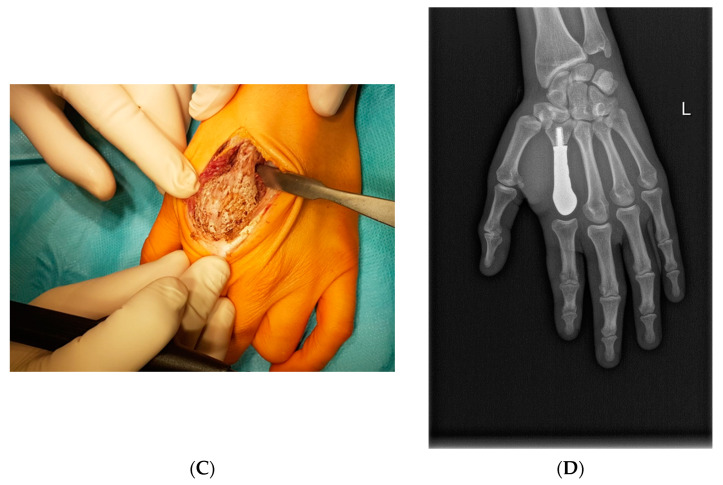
Response to denosumab therapy and radical resection of the GCTB of the second metacarpal followed by reconstruction with a 3D-printed patient-specific implant. (A) GCTB of the second metacarpal at the beginning of therapy; (B) X-ray taken after 13 doses of denosumab treatment; (C) Intraoperative image showing well-calcified tumor after dissection of surrounding soft tissue envelope; (D) 3D-printed patient-specific implant.
